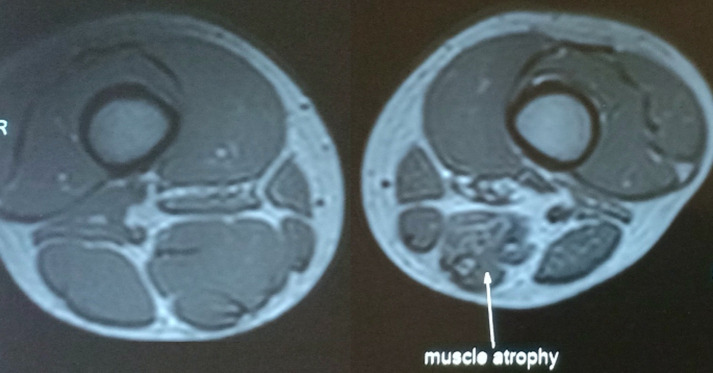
magnetic resonance imaging showing muscle atrophy
